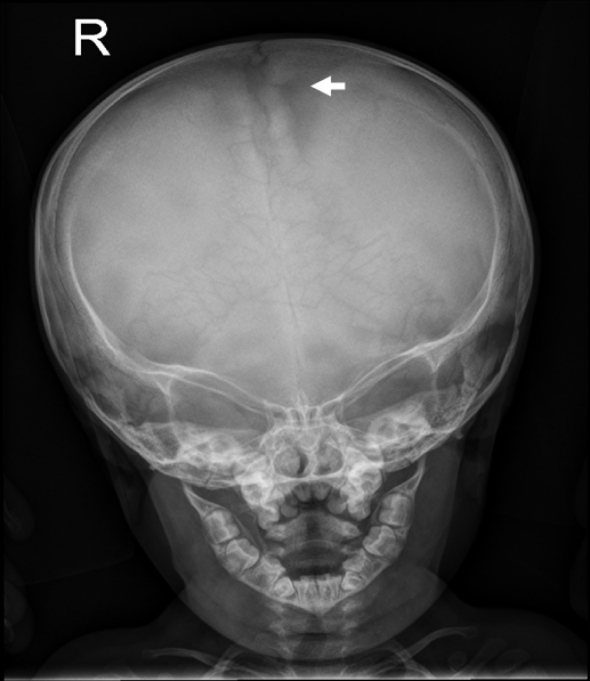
Anteroposterior skull radiograph showed brachycephaly, frontal bossing, defective ossification of the anterior fontanel (arrow), abundant wormian bones parallel to the sagittal suture and dental overcrowding of the upper jaw.
